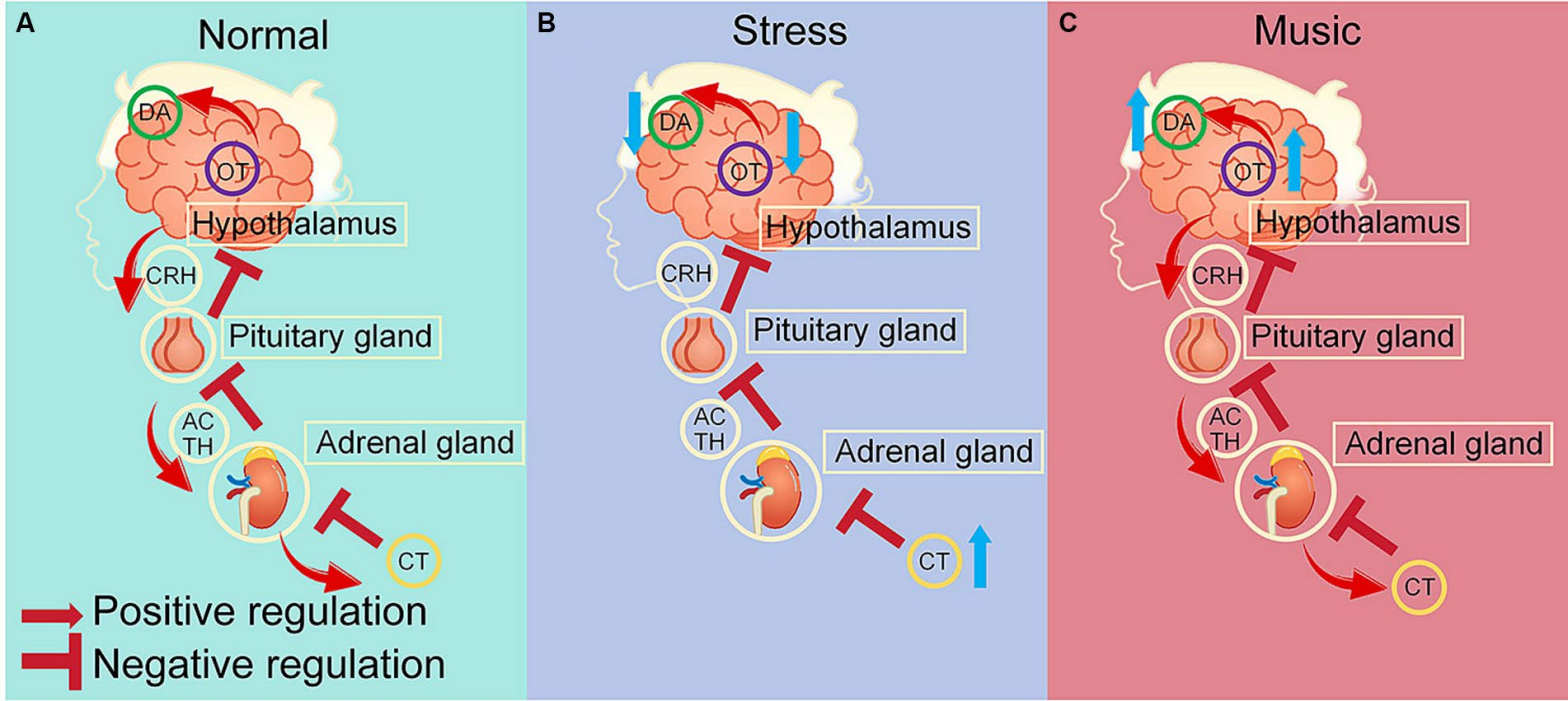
Neurotransmitter regulation by stress and acoustic stimuli. (A) Under normal conditions, dopamine, oxytocin maintain a dynamic equilibrium through interactions via the D2R and OTR receptors, oxytocin and cortisol maintain a reciprocal relationship via the hypothalamic-pituitary-adrenal (HPA) axis (Baskerville and Douglas, 2010; Tops et al., 2012; Love, 2014; Alley et al., 2019; Takayanagi and Onaka, 2022). (B) Under stress, increased cortisol levels trigger a negative feedback mechanism that downregulates the HPA axis and diminishes oxytocin levels, leading to downregulation of dopamine via the D2R-OTR interaction (Alley et al., 2019; Takayanagi and Onaka, 2022). (C) Musical stimuli normalize cortisol levels that are elevated due to stress, thereby restore the dynamic equilibrium of the HPA axis, and upregulate oxytocin, which in turn enhances dopamine levels through D2R-OTR interactions (Baskerville and Douglas, 2010; Tops et al., 2012; Love, 2014; Alley et al., 2019; Rasing et al., 2022; Xie et al., 2022; Okyay and Ucar, 2023). DA, dopamine; OT, oxytocin; CT, cortisol; CRH, corticotropin-releasing hormone; ACTH, adrenocorticotropic hormone.
